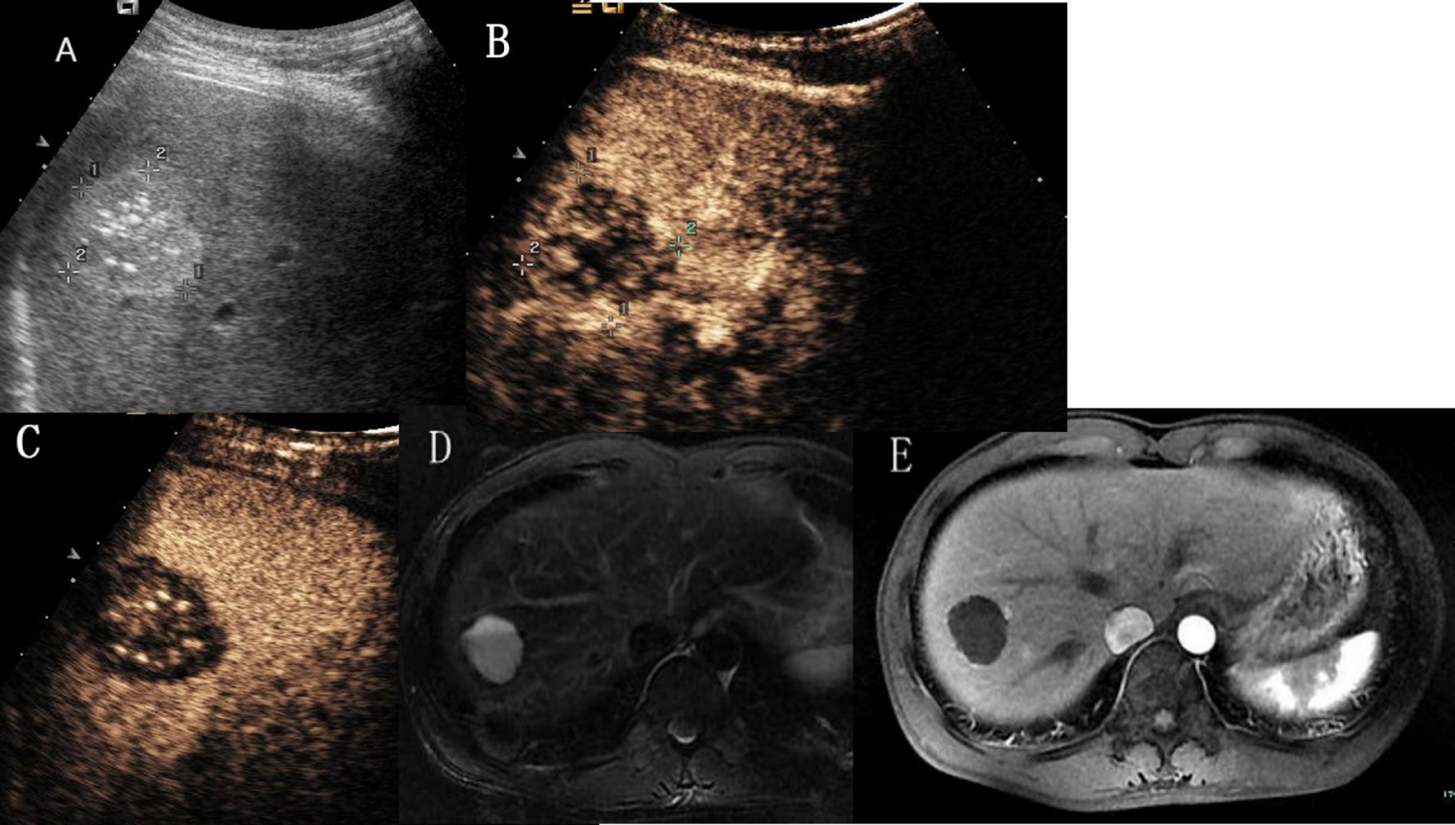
Hepatic hemangioma with no enhancement in all vascular phases (A) Ultrasound revealed a hyperechoic mass with multiple spotty calcifications in hepatic segment VII. (B) and (C) CEUS showed none enhancement throughout the whole vascular period. (D) T2- weighted MR images revealed a high signal intensity lesion with fluid–fluid level. (E) Enhanced magnetic resonance showed no contrast enhanced in arterial phase.
